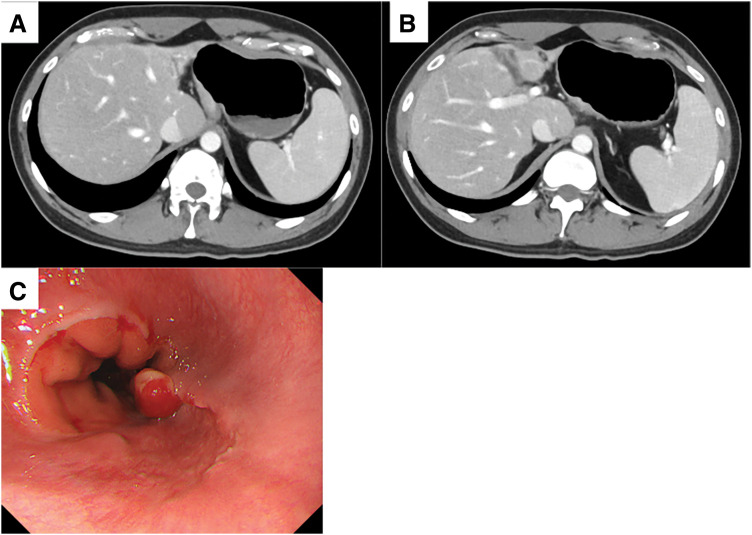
Contrast-enhanced CT and upper gastrointestinal endoscopy image before conversion surgery. (A, B) Multiple liver metastases and the portal vein tumor thrombus disappeared, and gastric wall thickening and lymph node swelling also disappeared. (C) Upper gastrointestinal endoscopy revealed areas of scarring due to treatment and elevated lesions suggestive of residual cancer.
